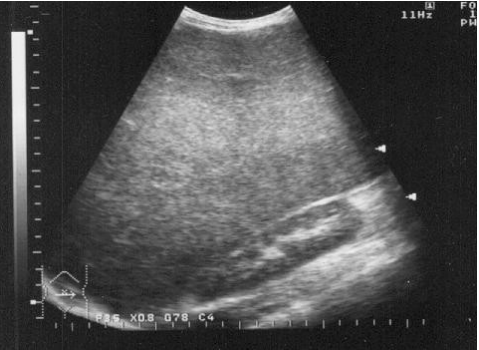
Longitudinal sonogram of liver showing hepatomegaly and increased parenchymal echogenicity with posterior shadowing (*) compatible with fatty infiltration.
